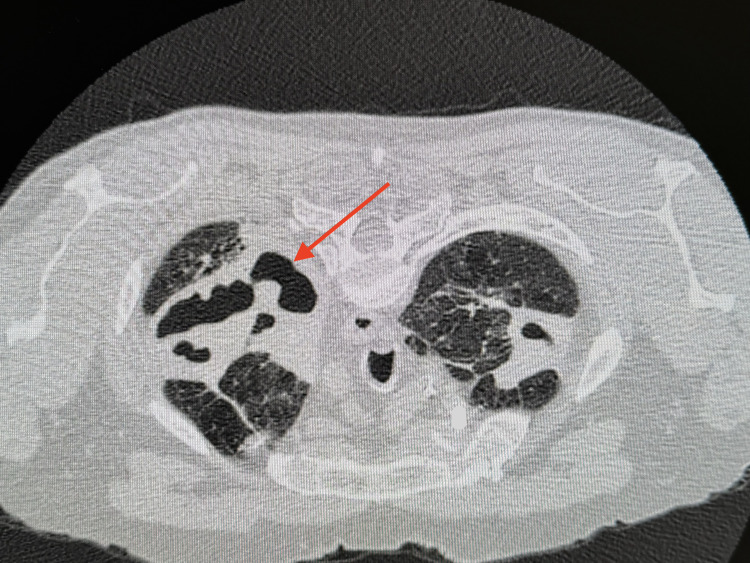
Axial sections of chest CT with contrast that show large areas of cavitation within the upper lobes of the lungs (arrow) CT: computed tomography
